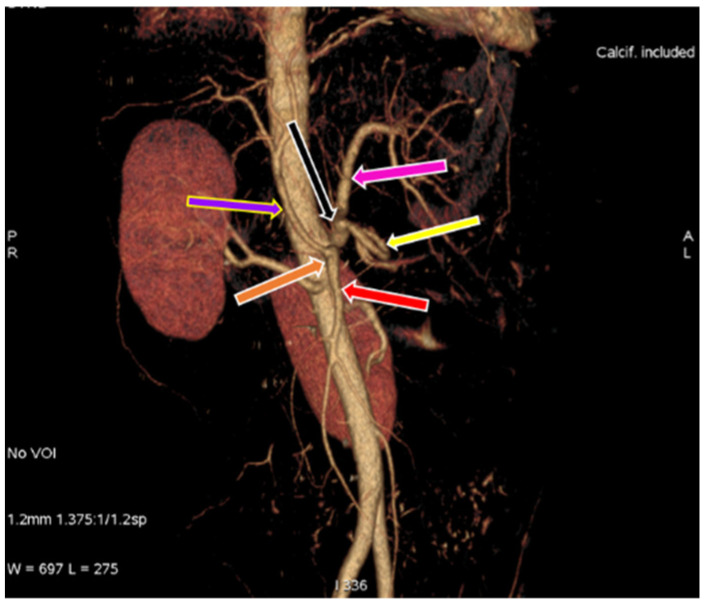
3D-VR image of (64 row scanner CT angiography) abdominal region showing gastrosplenic (black arrow) and hepato-mesenteric trunk (orange arrow), gastrosplenic trunk divides into left gastric artery (pink arrow) and splenic artery (yellow arrow), hepato-mesenteric divides into common hepatic artery (purple arrow) and superior mesenteric artery (red arrow).
